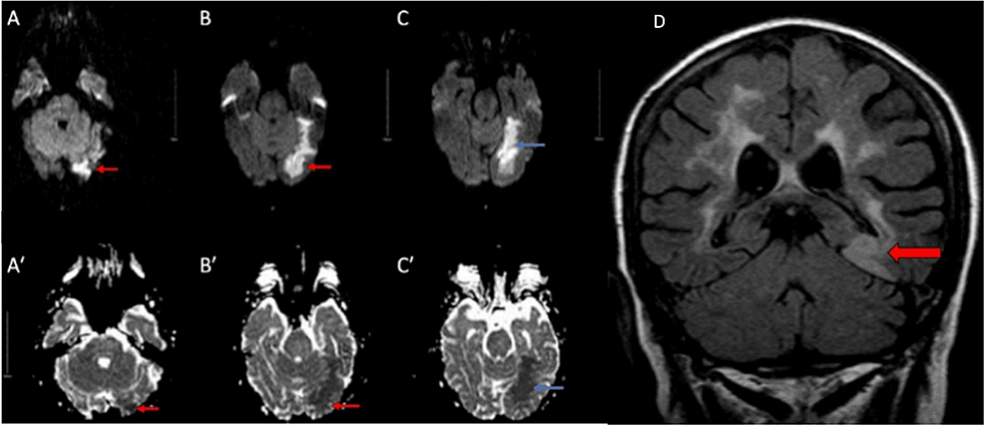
Case 1: Acute left PCA stroke (A-C’) Superior row, MRI DWI sequence. Inferior row, MRI apparent diffusion coefficient (ADC) sequence. The image shows matched DWI and ADC signals in the occipital (red arrows) and medial temporal lobes (blue arrows), consistent with acute cerebral infarction. (D) T2 FLAIR view shows ischemic lesions to the left parahippocampal gyrus and fibers adjacent to the lateral ventricle (red arrow). DWI, diffusion-weighted imaging; FLAIR, fluid-attenuated inversion recovery; PCA, posterior cerebral artery
